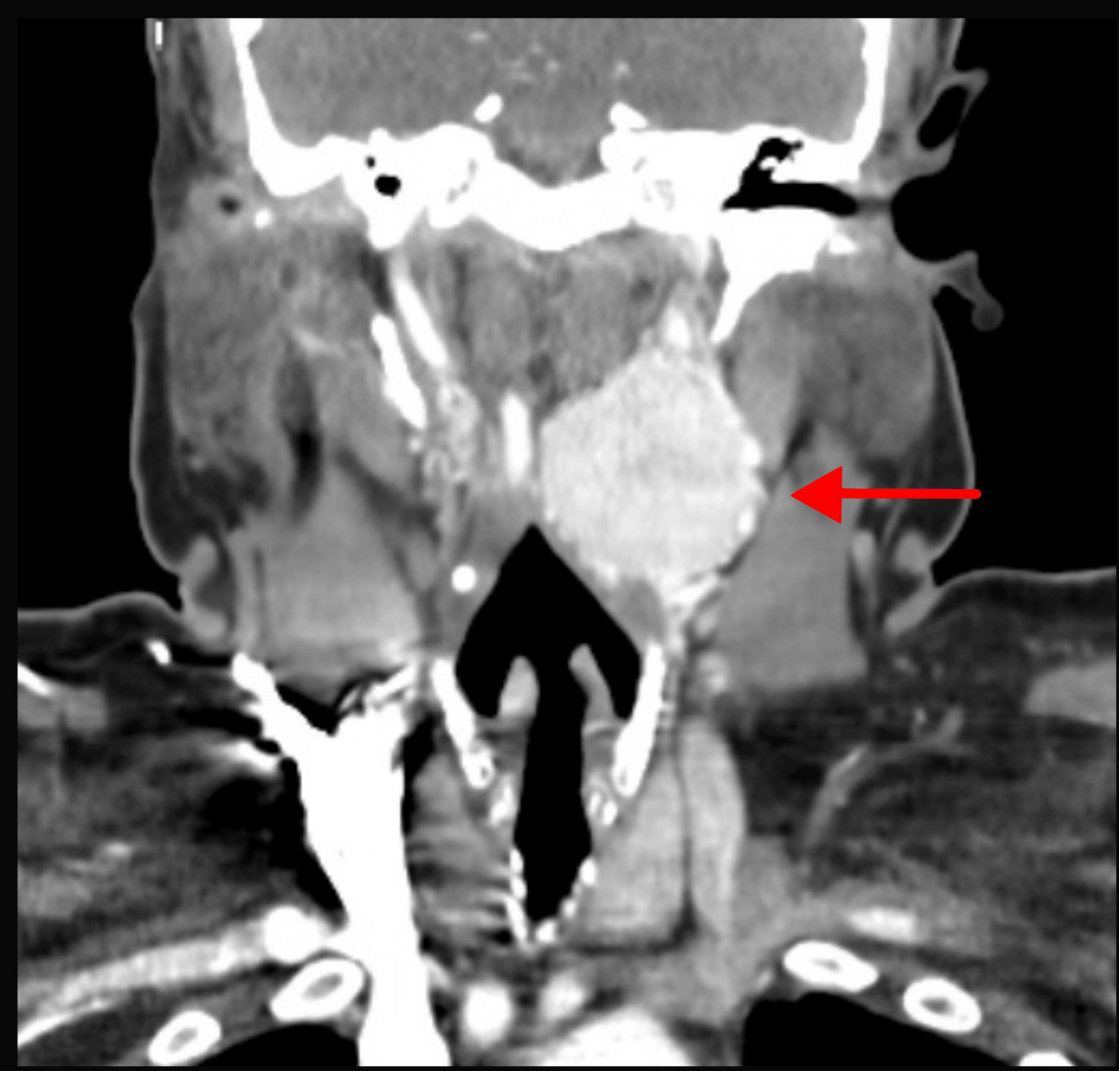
CT angiography showing a coronal image of a Shamblin type III CBT CBT, carotid body tumor
